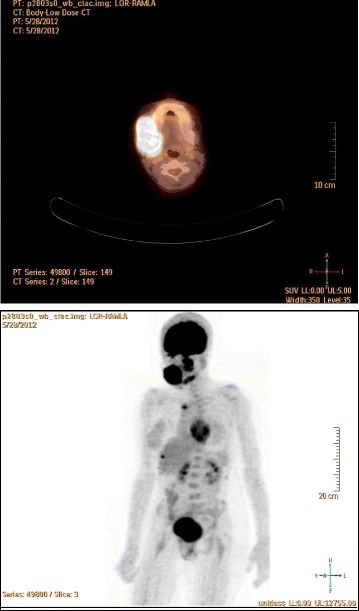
Positron emission tomography computed tomographic scan showing multiple hypermetabolic, expansile, bony lytic lesions in the right mandible, left maxillary sinus, left mandible, and manubrum sterni
